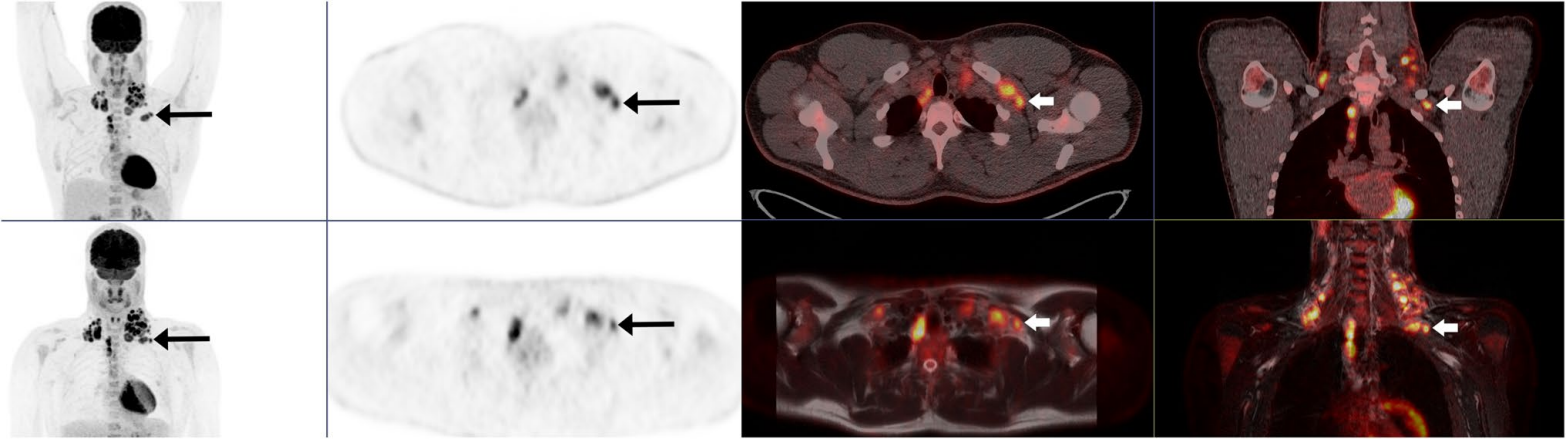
A 29-year-old male with classical HL stage IIB. Baseline PET/CT (arms up) in the upper row and PET/MR (arms down) in the bottom row. In consensus PET/MR, the left infraclavicular FDG avid lymph node was classified as left cervical, but scored as left infraclavicular on PET/CT
